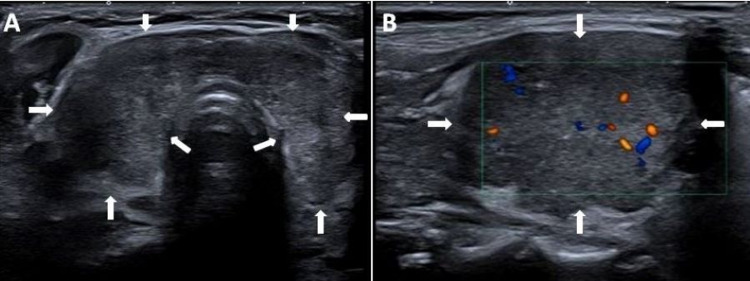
Ultrasound scan of the thyroid gland Enlarged thyroid gland with heterogeneous echotexture (A), and Doppler studies of right thyroid lobe showing reduced vascular flow (B).
